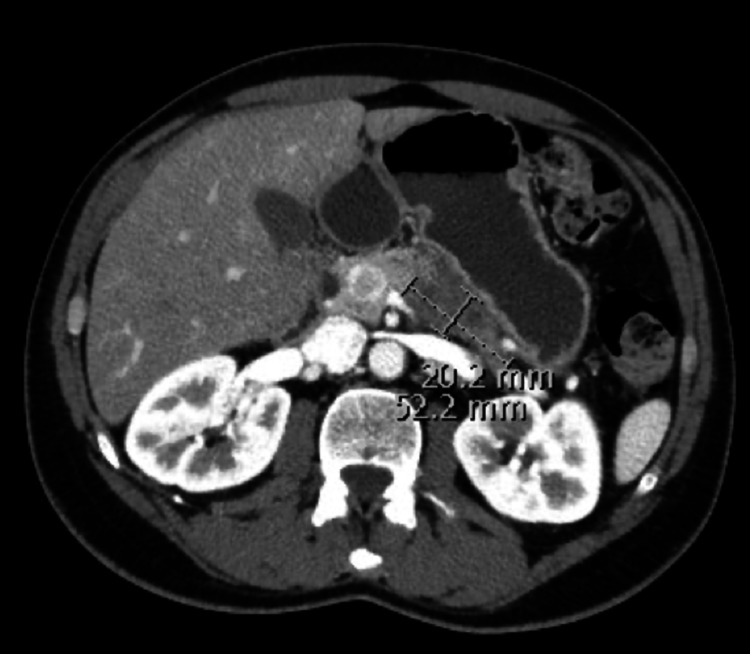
CT abdomen shows pancreatic mass prior to starting second-line GNP chemotherapy (Case 3) Arrows indicate the pancreatic mass
